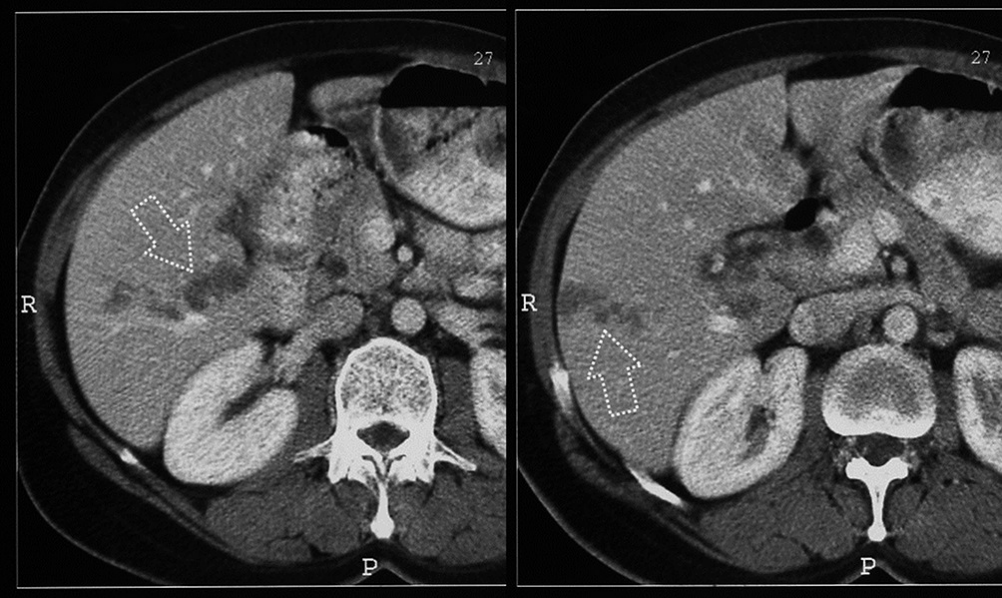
Contrast-enhanced CT images in the portal phase demonstrate two hypodense curvilinear tracts, extending from the liver capsule to the parenchyma, in the hepatic segments V and VI (arrows).
